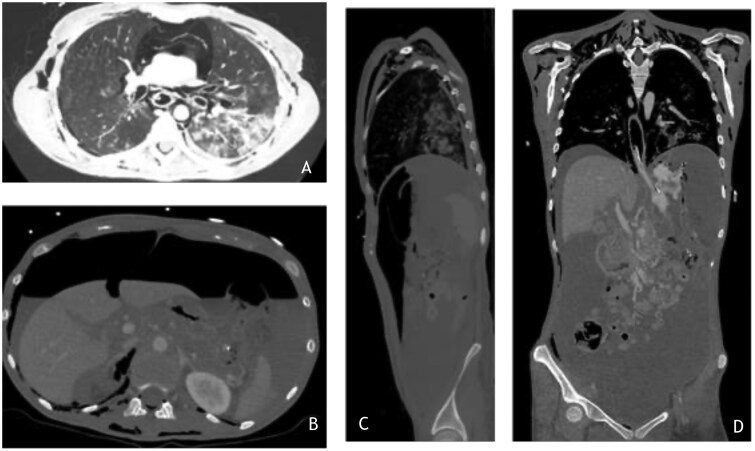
(A) Chest CT in a lung widows demonstrating subcutaneous emphysema, a tiny right pneumothorax and pneumomediastinum. In the left lung base consolidation due to aspiration. (B) Abdominal image showing extensive free air and peritoneal fluid, due to the ischaemic stomach blowout. (C) Sagittal reconstruction of the CT-abdomen again showing the free air, subcutaneous emphysema and the aspiration pneumonia. (D) coronal reconstruction partially depicting the nasogastric tube and the free peritoneal fluid, as well as pneumatosis in the fornix of the stomach.
